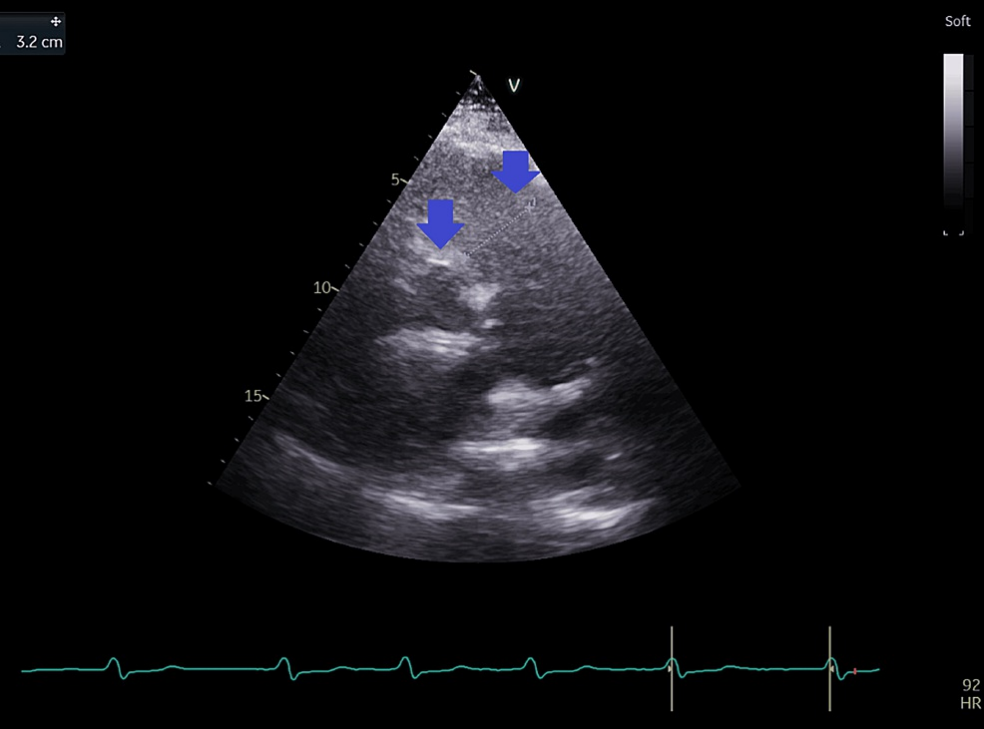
Short-axis view at the base of the heart from the left parasternal window with measurement of the right ventricular outflow tract diameter at 3.2 cm (blue arrows).
